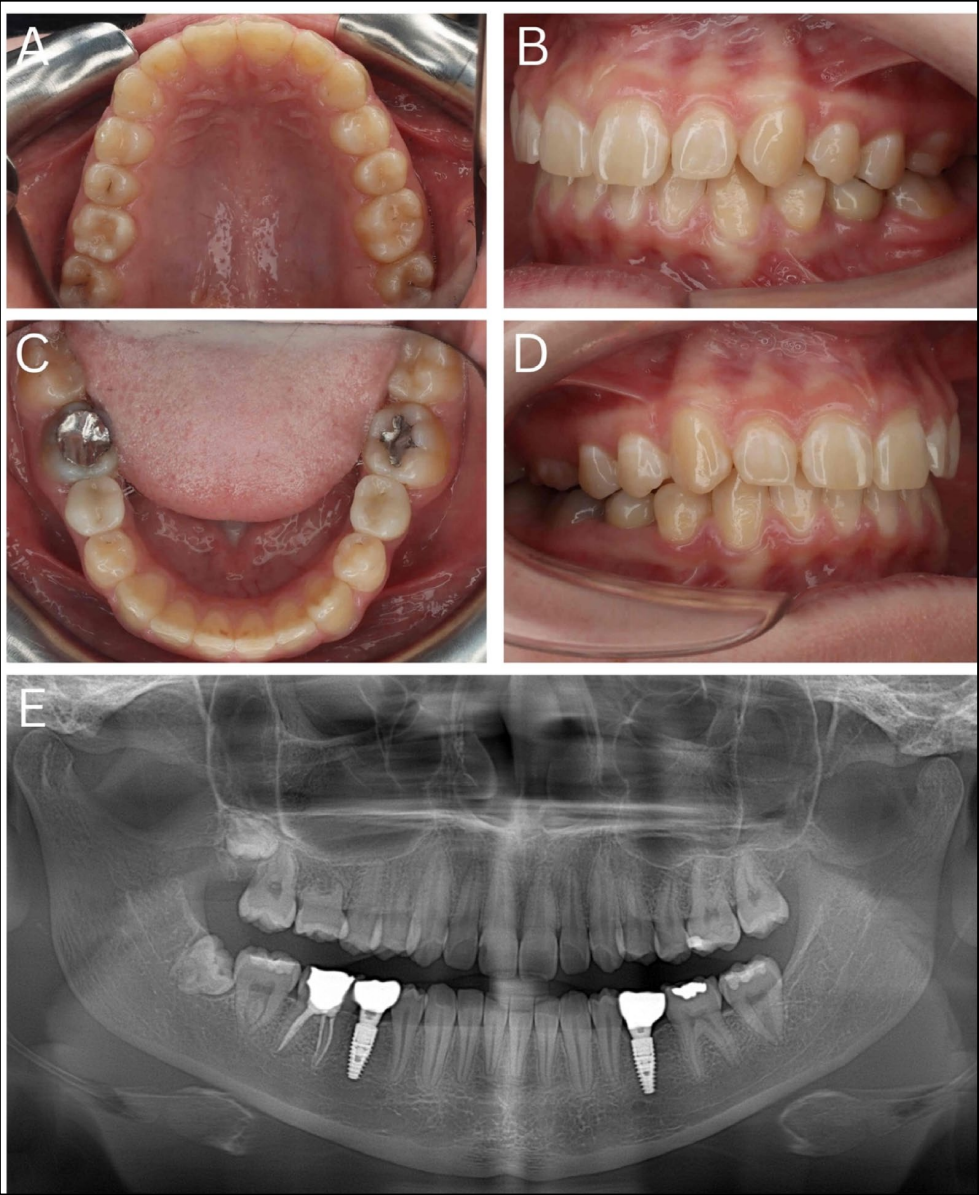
Clinical and radiographic follow-up 2 years after implantation and 3 years after autotransplantation. A Occlusal view of the maxillary arch demonstrating stable occlusion and integration of the autotransplanted tooth at site 16. B, D Right and left lateral intraoral views showing well-aligned occlusion and healthy gingival tissues around the autotransplanted and implant-supported restorations. C Occlusal view of the mandibular arch showing implant-supported crowns in regions 35 and 45, with healthy peri-implant tissues. E Panoramic radiograph taken 2 years after implantation and 3 years after autotransplantation showing stable implant osseointegration and transplanted tooth function; third molars (teeth 18 and 48) were later extracted for micro-computed tomography analysis of therapy-related changes
